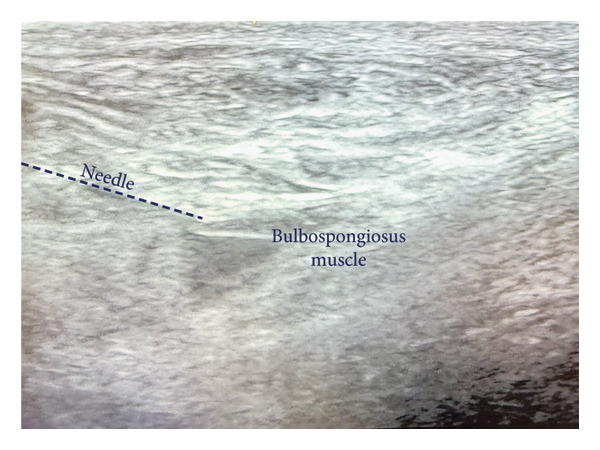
Ultrasound‐guided TPI of the bulbospongiosus muscle, alternate view.
